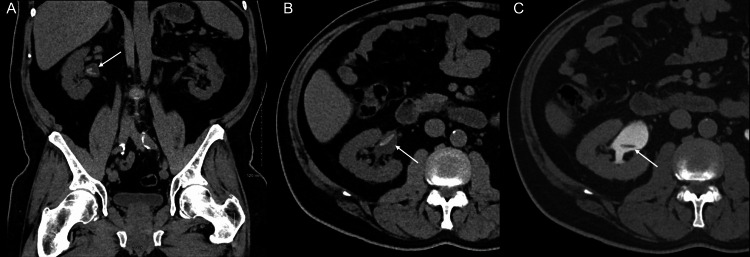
Coronal (A) and axial (B) non-contrast CT images in soft tissue window through the right kidney revealed a 2.6 x 0.5 cm tubular-shaped calcification within the right renal pelvis (white arrow). Axial delayed contrast CT (C) also through the right mid kidney demonstrated a corresponding dependent filling defect in the contrast filled renal pelvis (white arrow).
